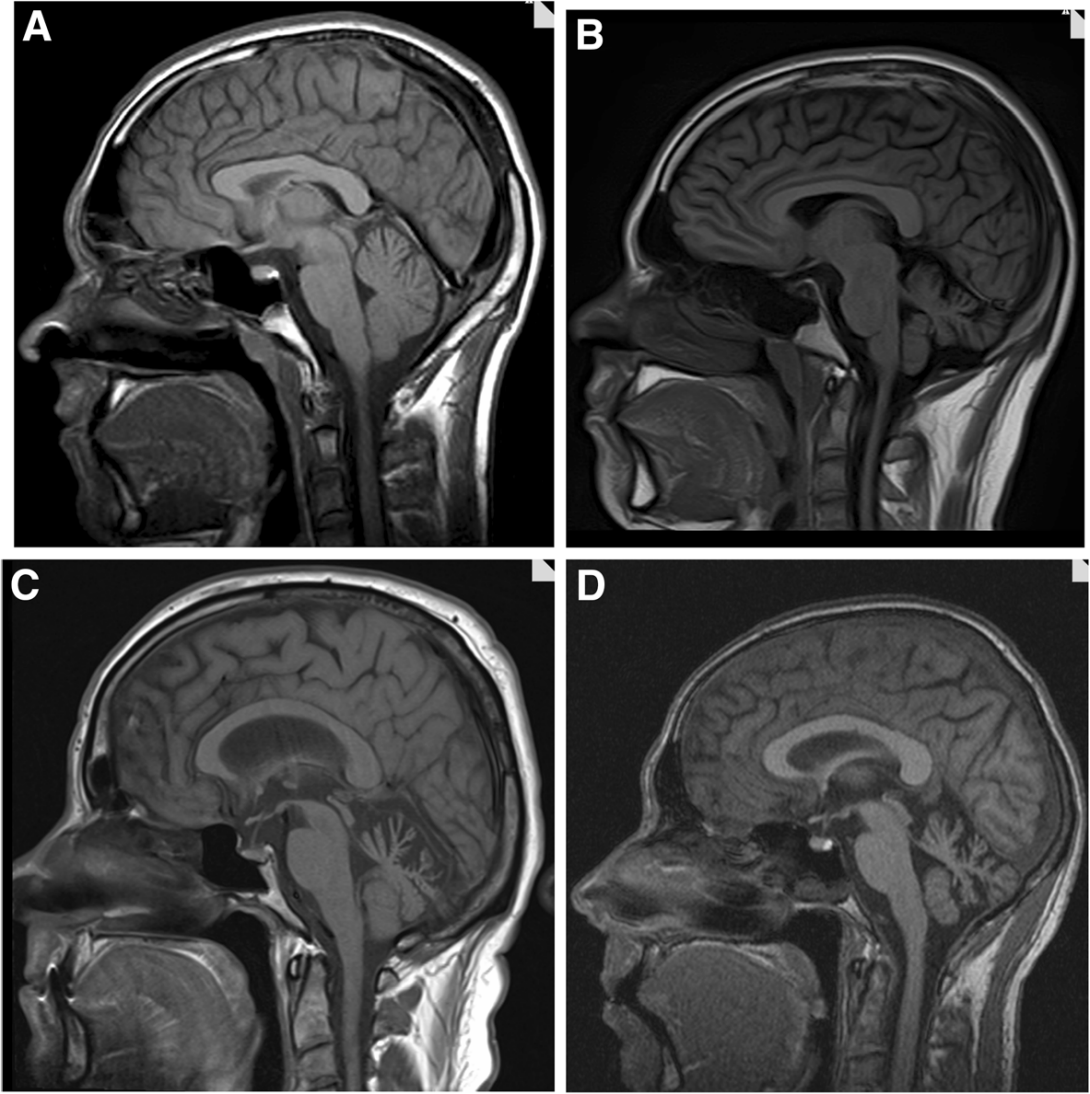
MRI from four SCA14 subjects at time of neuropsychological testing. A: Subject VI-2, disease duration 7 years; B: Subject VI-3, disease duration 10 years; C: Subject III-3, disease duration 18 years; D: Subject V-3, disease duration 25 years.
